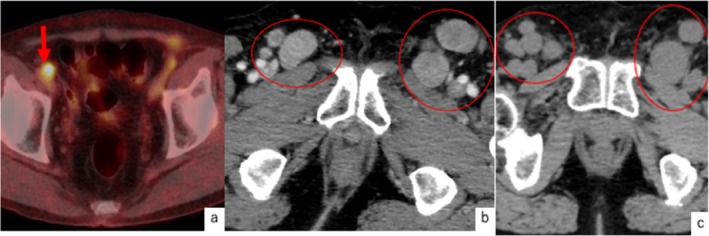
FDG‐PET at metastatic diagnosis (a), CT before chemotherapy (b), and after chemotherapy (c).
